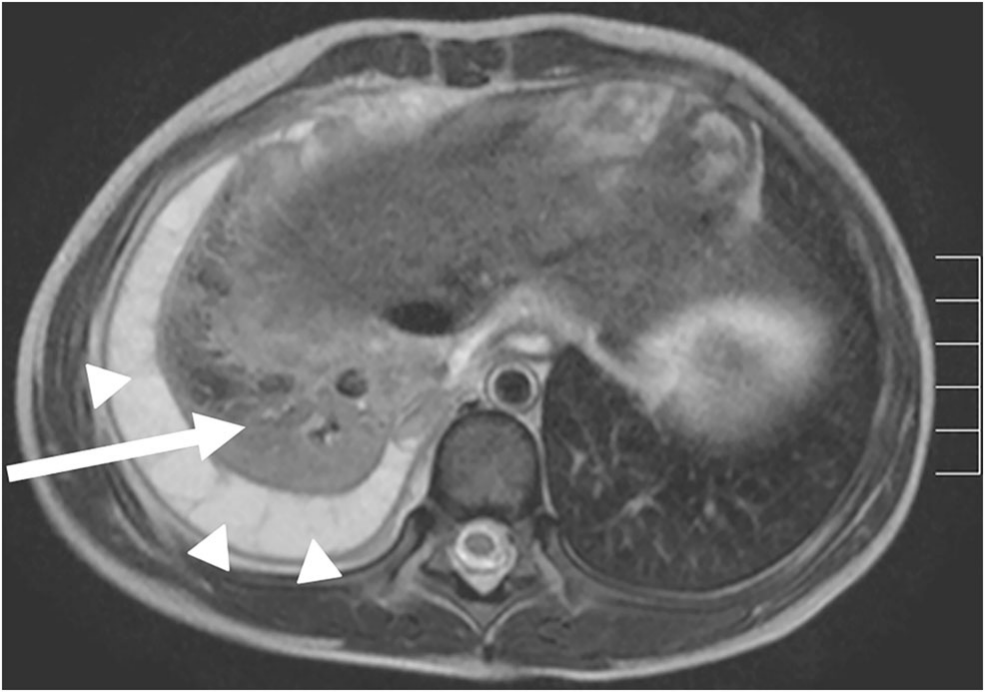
Pneumonia on lung MR in a 5-year-old girl (axial T2-weighted turbo spin echo, parameters in Table 2). There is almost complete alveolar infiltration in the right lower lobe (*arrow*) caused by lobar pneumonia. Note pleural effusion with fibrinous septation (*arrowheads*)
